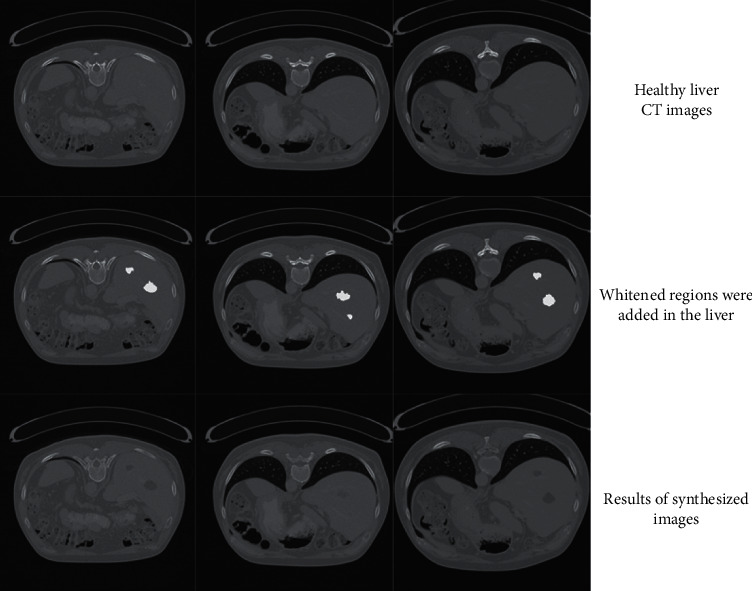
Adding tumor regions on the healthy liver CT images and synthesizing diseased liver CT images using our method.
